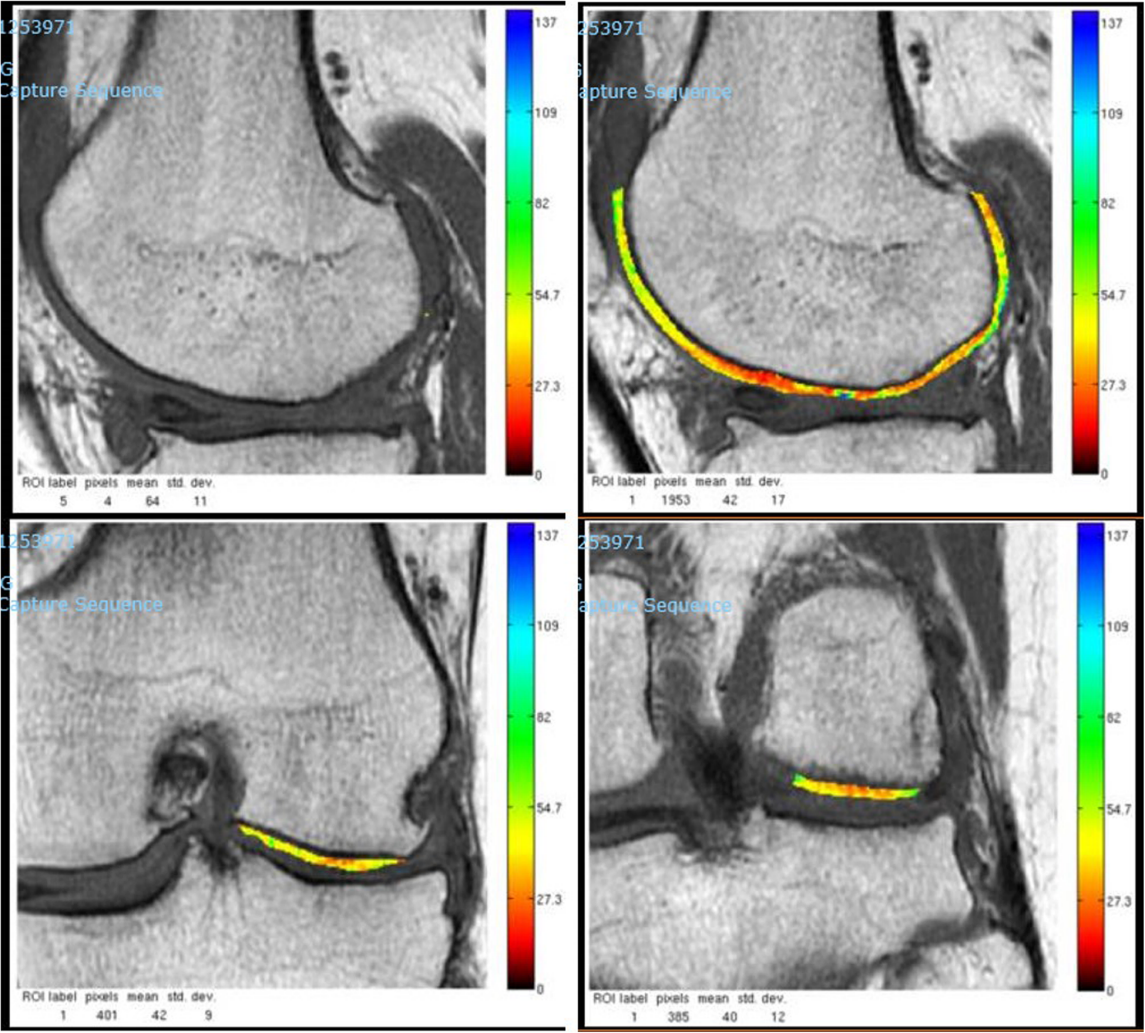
MRI of the same patient at 1 year follow-up. The T2 cartilage maps showed a full-thickness coverage of the defect with a heterogeneous and irregular tissue. The superficial layer of the tissue seems to have a higher content of water, while the layer close to the subchondral bone shows a structure more similar to normal cartilage. There is no bone edema in the area of the scaffold
